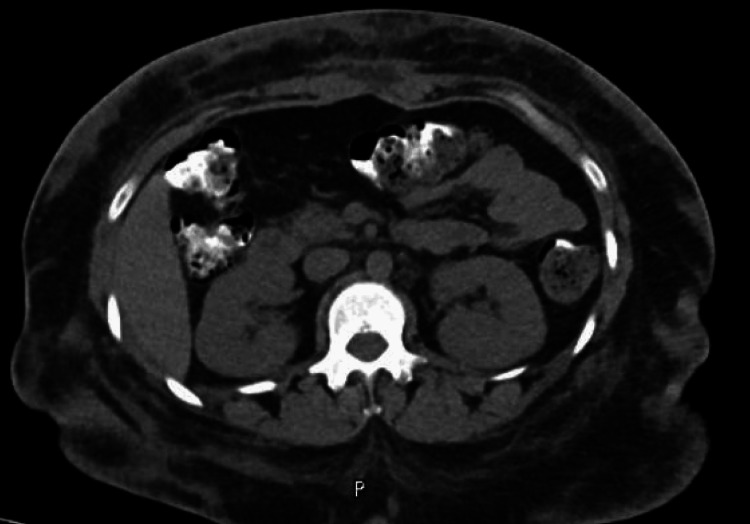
CT showed re-accumulation of access and necrotic tissue with classification and post-operative changes
